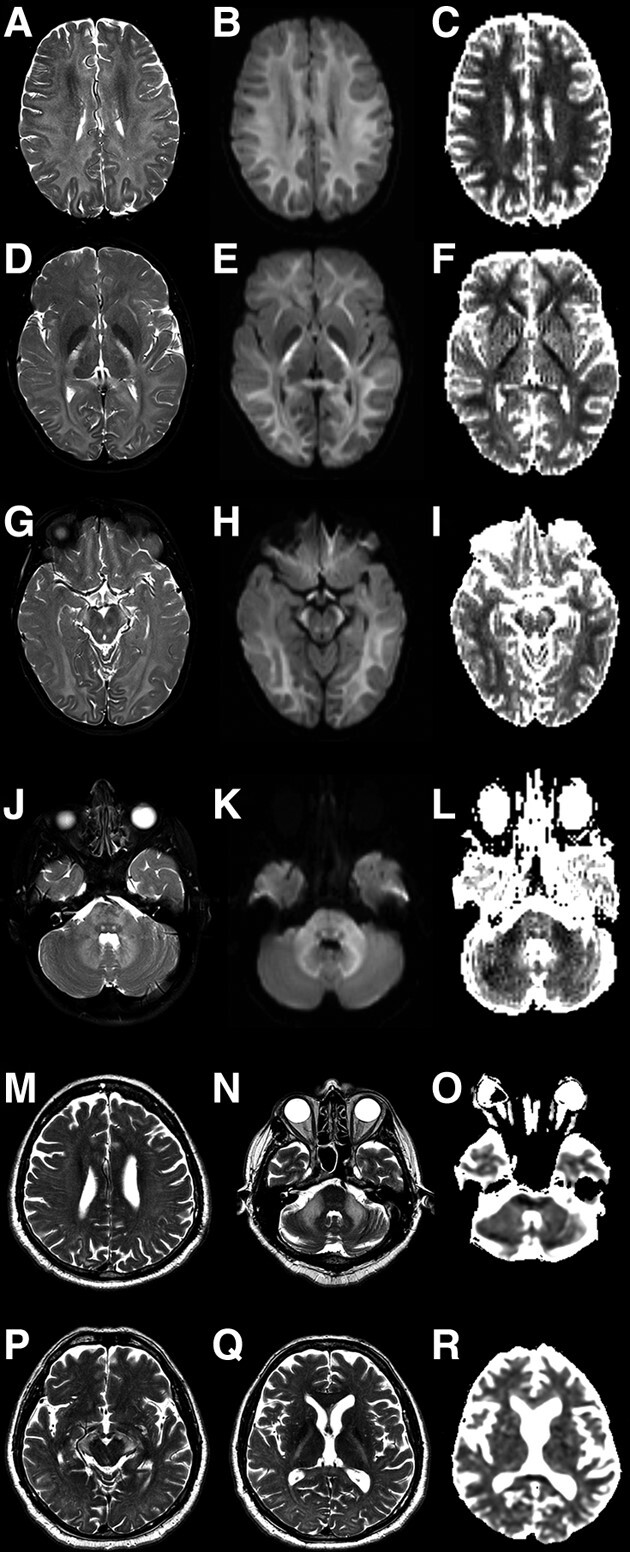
MRI findings in patients with *CLCN2-*related leucoencephalopathy and ataxia. Brain MR from patient 3 (A–L). Axial T2-weighted images (A, D, G, J) demonstrate symmetric bilateral hyperintensity involving the cerebral and cerebellar white matter, posterior limb of internal capsules, midbrain cerebral peduncles, central tegmental tracts (arrowheads in G) and middle cerebellar peduncles. The corresponding DWI (B, E, H, K) and ADC maps (C, F, I, L) suggest restricted diffusion, manifest as DWI hyperintensity and ADC map hypointensity, bilaterally in the subcortical cerebral white matter (arrows in C), posterior limb of internal capsules (arrows in F), midbrain cerebral peduncles (arrows in I) and middle cerebellar peduncles (arrows in L). Brain MR from patient 1 (M–R). Axial T2-weighted images (M, N, P, Q) show symmetric bilateral mild hyperintensity involving the cerebral white matter, which is more prominent in the subcortical region of the right parietal lobe (arrow in M); there is also hyperintensity in the posterior limb of internal capsules, midbrain cerebral peduncles, central tegmental tracts (arrowhead in N) and middle cerebellar peduncles. Also notice the compromise of the splenium of the corpus callosum (arrow in Q). The corresponding ADC maps (O, R) demonstrate hyperintensity in the posterior limb of internal capsules and middle cerebellar peduncles (arrows in O), which represents facilitation (not restriction) of the movement of water molecules. There is some restriction in the splenium of the corpus callosum, shown as hypointensity in the ADC map (arrowhead in R).
